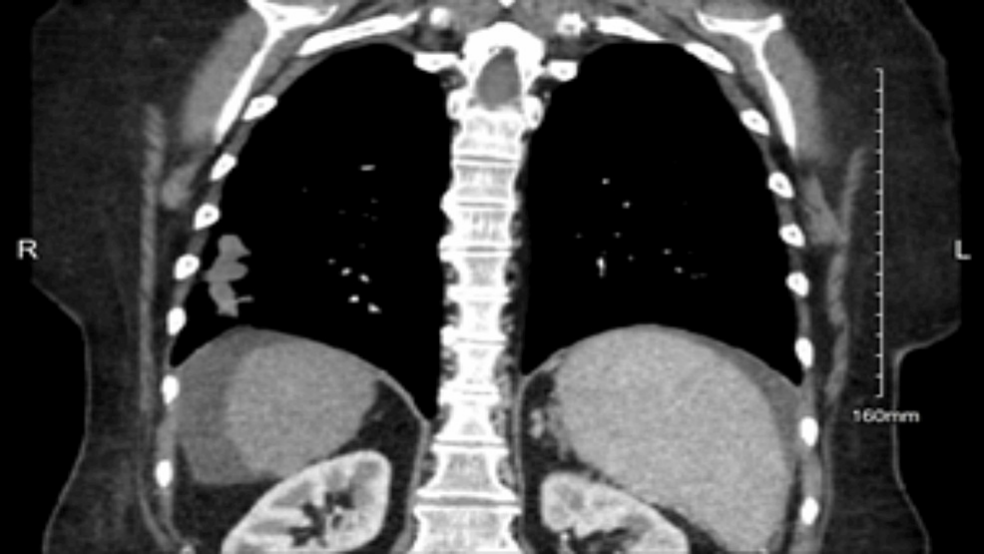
CT scan of the abdomen and pelvis with IV and oral contrast. Multiple pulmonary nodules and masses in the right middle and lower lobe of the lungs.
